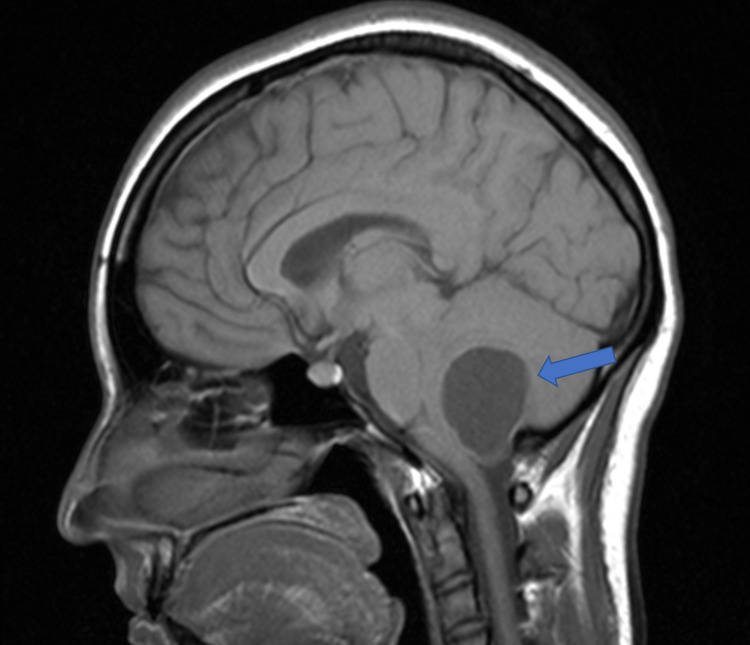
Sagittal T1 MRI without contrast Cystic lesion in the cerebellar hemisphere with associated mass effect and compression of the fourth ventricle and dorsal brainstem (blue arrow)
